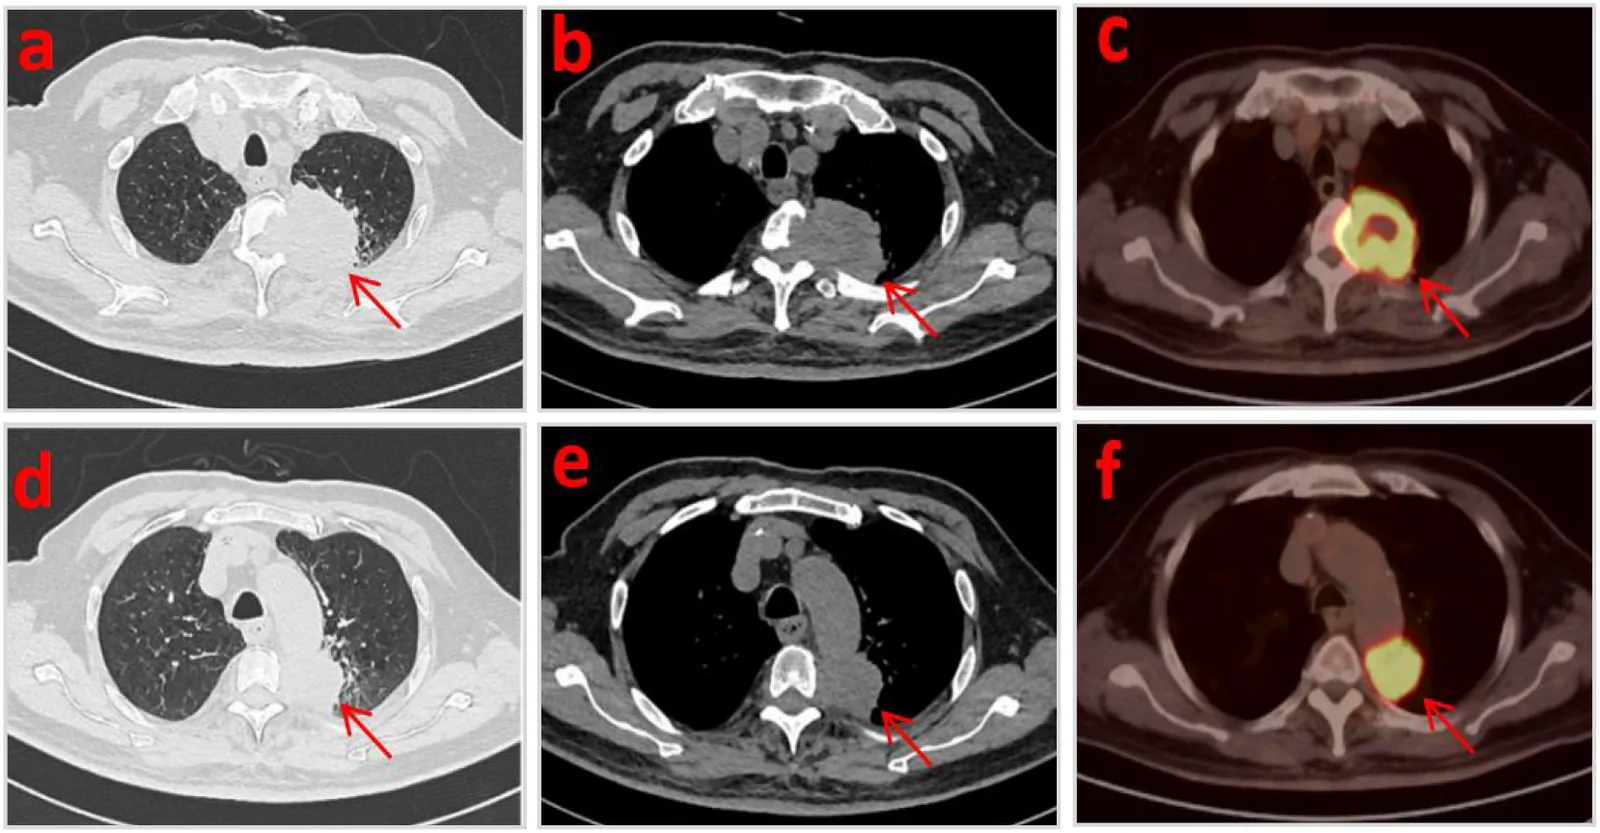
CT and FDG-PET/CT presentation of a T4 left upper lung cancer. The image shows a tumor from the upper left lung under lung window (a, d) mediastinum window (b, e) and FDG-avid on PET-CT (c, f). CT scans (a–c) demonstrate extensive invasion of the adjacent vertebrae bodies with tumors occupying almost 50% of a vertebrae body.
